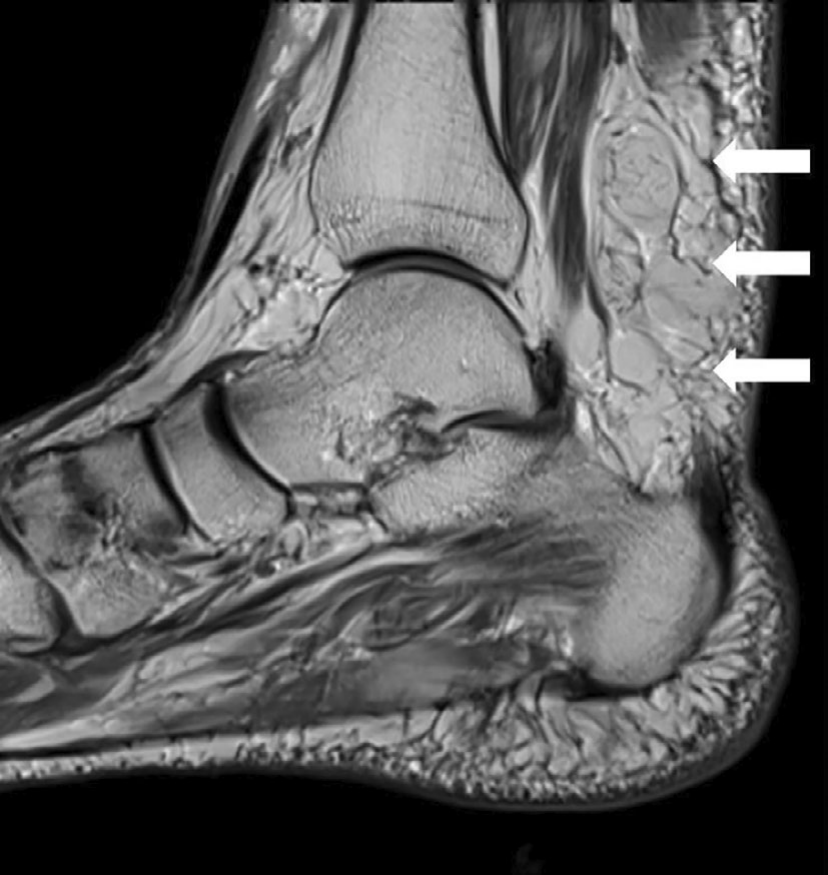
Sagittal plane of T1‐weighted MRI showed significant enlargement of the Achilles tendon (diameter ~ 2.8 cm) extending from the calcaneus into the musculotendinous junction, suggestive of xanthoma infiltration.
